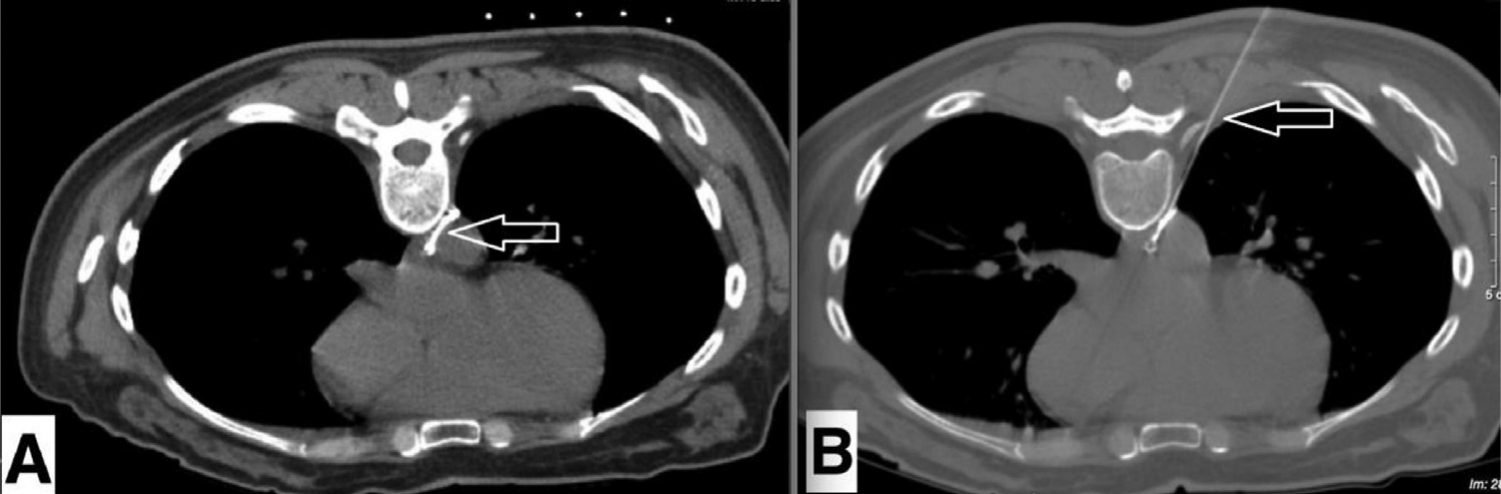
Chest CT scans 8 days after thoracic duct (TD) embolism. A) The TD, containing contrast agent, was observed (arrow). B) A needle was used to puncture the TD under CT guidance (arrow), and the sclerosing agent was injected into the TD.
